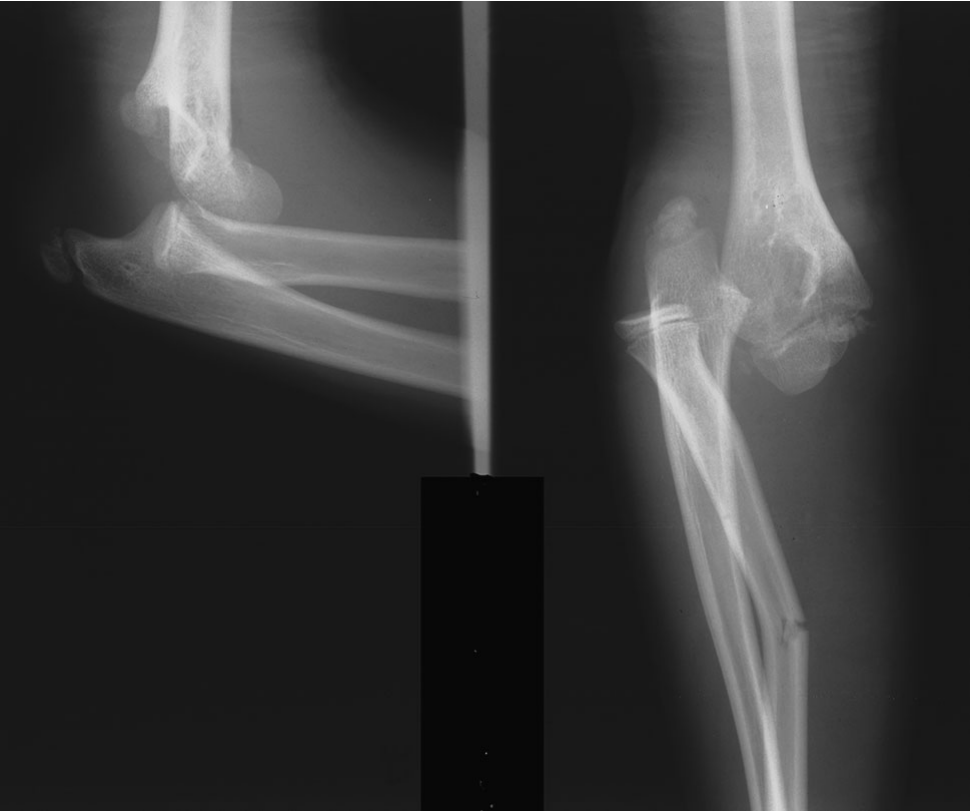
Radiographs of a 13-year-old boy with a type II segmental injury of the radius. It combined a dislocated elbow and a diaphyseal fracture of the radius
